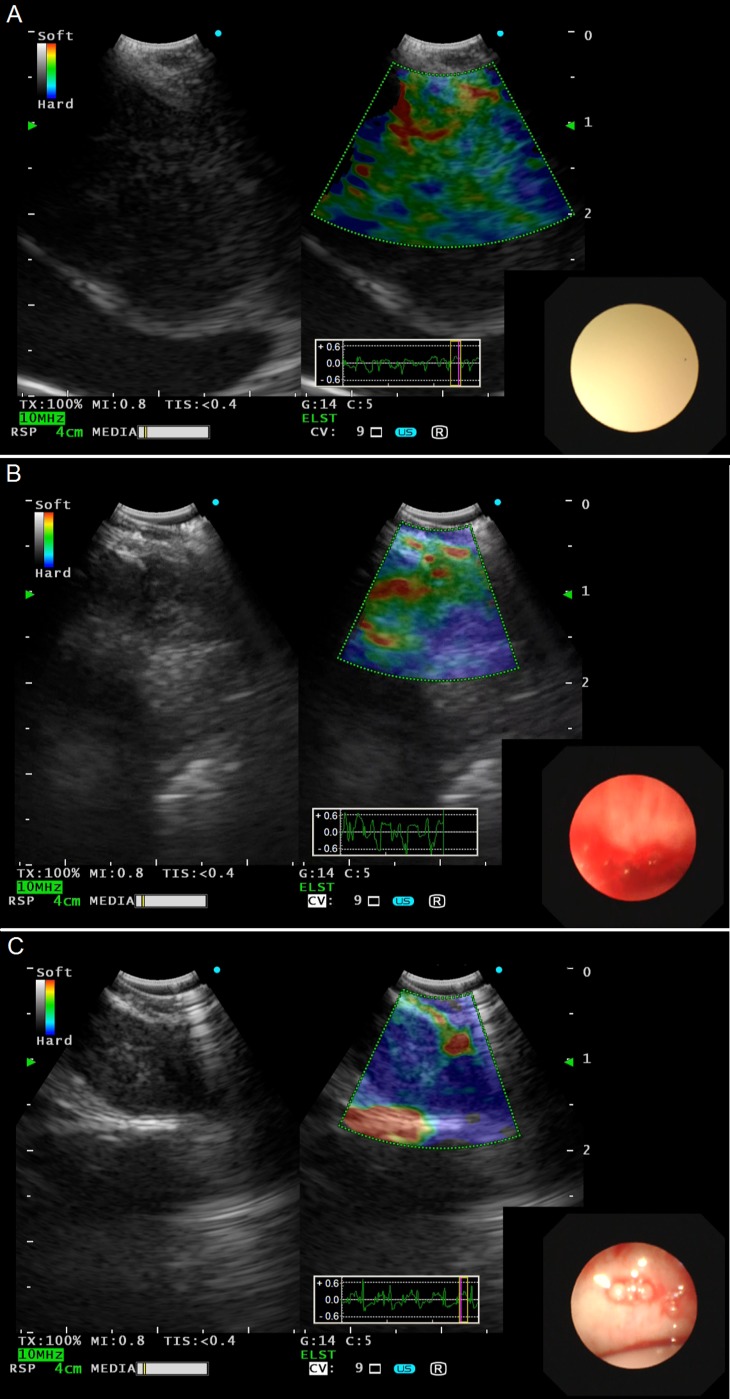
Representative lymph nodes on EBUS elastography A. Type 1: dominant colors were green, yellow or red. B. Type 2: part blue, part green, yellow or red. C. Type 3: dominant color was blue. EBUS, endobronchial ultrasound.
